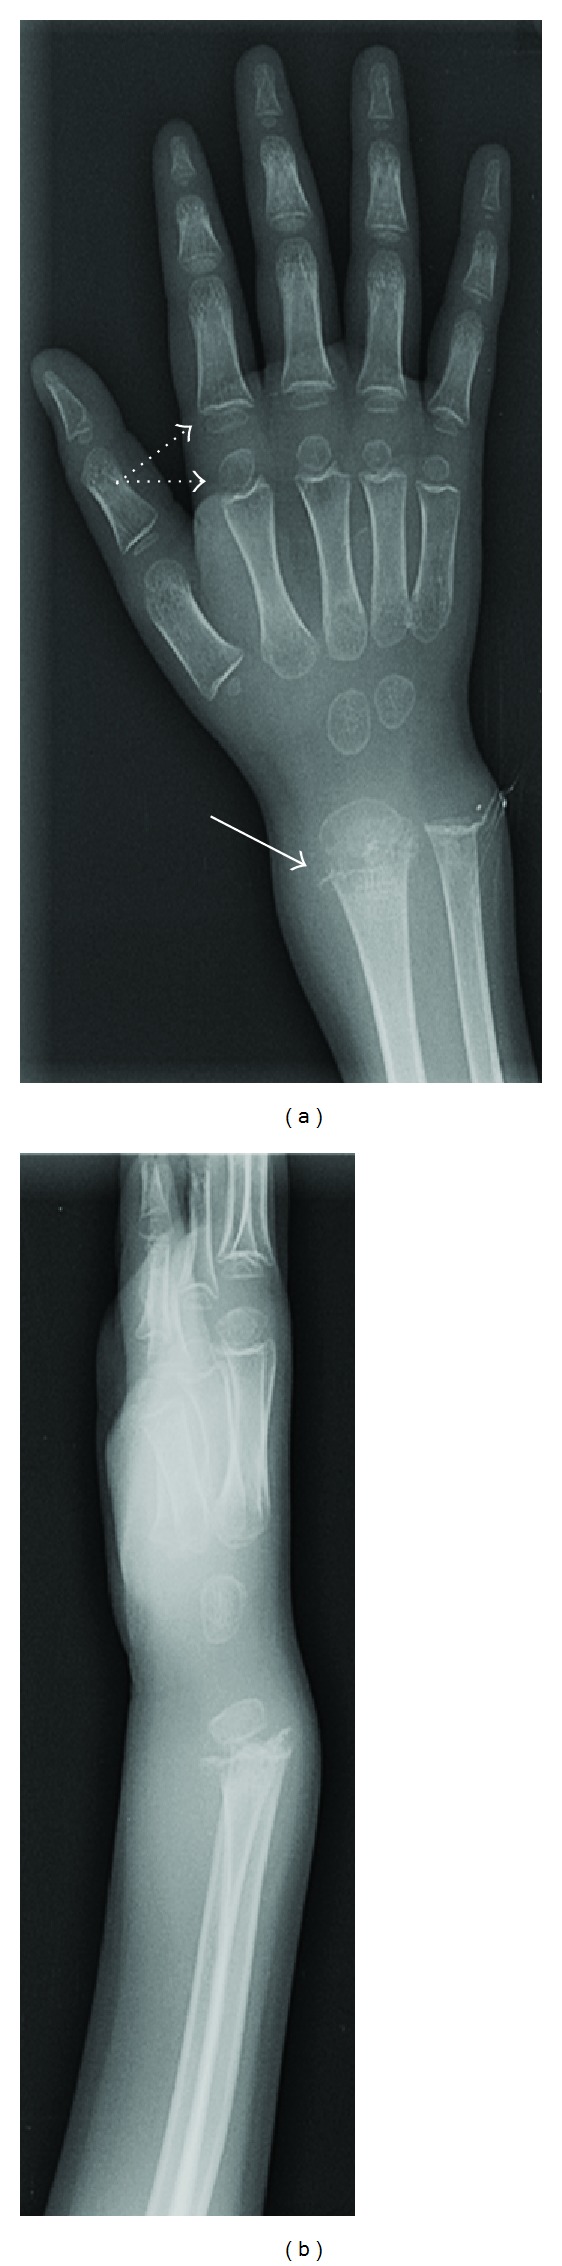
Anteroposterior (a) and lateral (b) X-rays of the hands and wrists showing severe osteopenia of the epiphyses of the phalanges (dotted arrow), metacarpals and the carpal bones, epiphyseal separation of the distal radius and ring sign (arrow).
